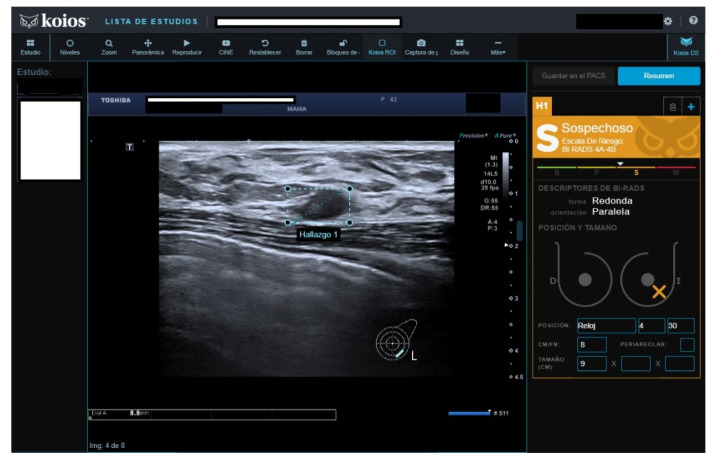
Nodule rated B3 by reader (no Doppler), KSS by KOIOS. Cytology reported grade 1 carcinoma. Biopsy confirmed invasive carcinoma.
